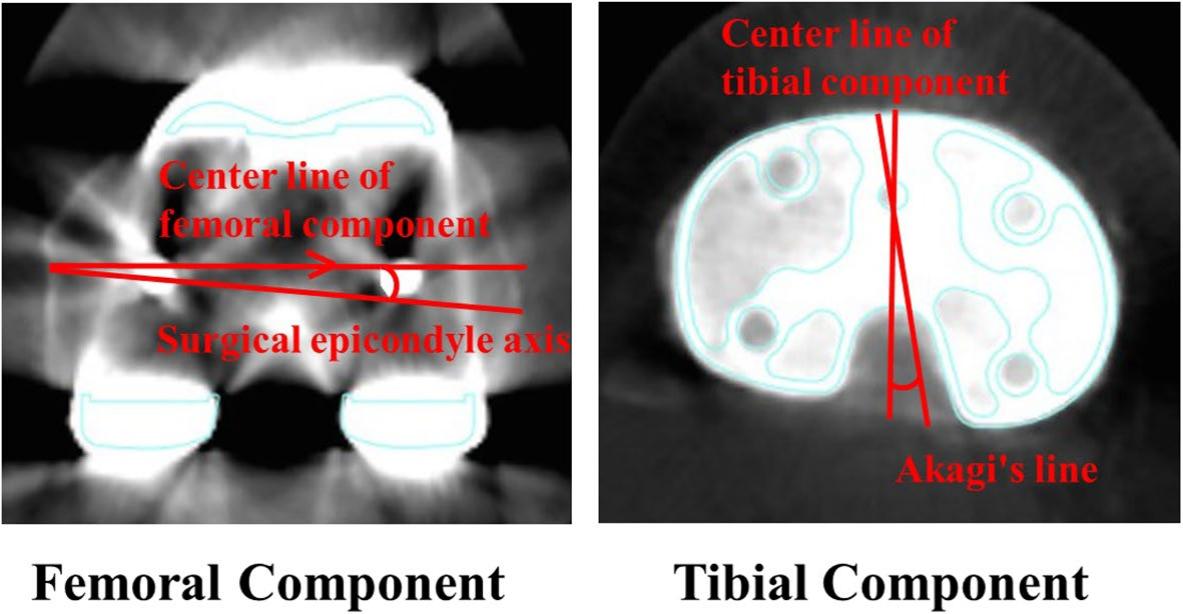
Femoral and tibial component rotational angle. Axial computed tomography (CT) image of the femur. The surgical epicondylar axis (SEA) connects the lowest point of the medial epicondyle to the midpoint of the lateral epicondyle. The prosthetic posterior condylar line (PCL) connects the medial and lateral prosthetic posterior condylar surfaces. The femoral component rotational angle was defined as the angle between the SEA and the PCL. Axial CT image of the tibia. Akagi’s line connects the center of the posterior cruciate ligament and the medial border of the tibial tuberosity. The tibial component rotational angle was defined as the angle between the centerline of the tibial component and Akagi’s line. The difference in rotational angles between the femoral and tibial components constituted the rotational mismatch
